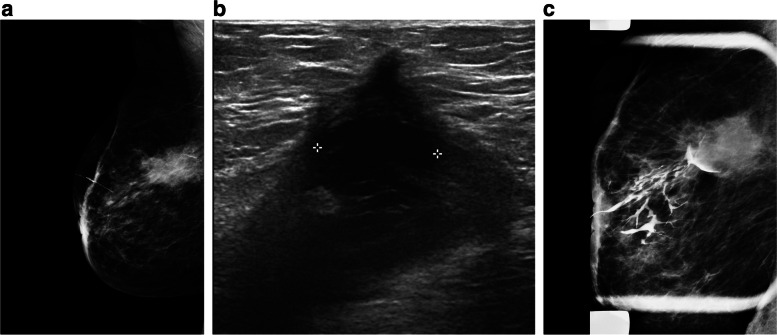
A 82-year-old woman with a history of resected DCIS in the right breast. She presented with a 3-week history of serous sanguineous spontaneous nipple discharge. There were post-surgical changes in the right upper outer quadrant, including the known seroma, which appeared slightly decreased overall compared to previously. The right breast ultrasound did not show any suspicious lesion. In ductography, multiple ducts were opacified and extended into the upper outer breast towards the lumpectomy site with a cut-off at the seroma site. No filling defect within the ducts was identified. The nipple discharge was suspected to be coming from a seroma secondary to fistulization of the seroma within the ducts. A short-term follow-up US was recommended. After a few months, the discharge stopped
